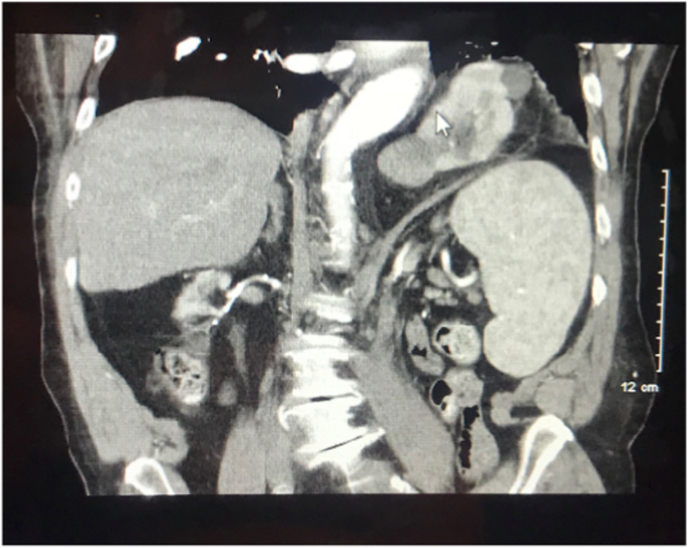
CT of the abdomen shows an intrathoracic left kidney within left diaphragmatic hernia and an intermediate density lesion arising from the anterior mid left kidney measuring 3.9 × 3.6 cm.
